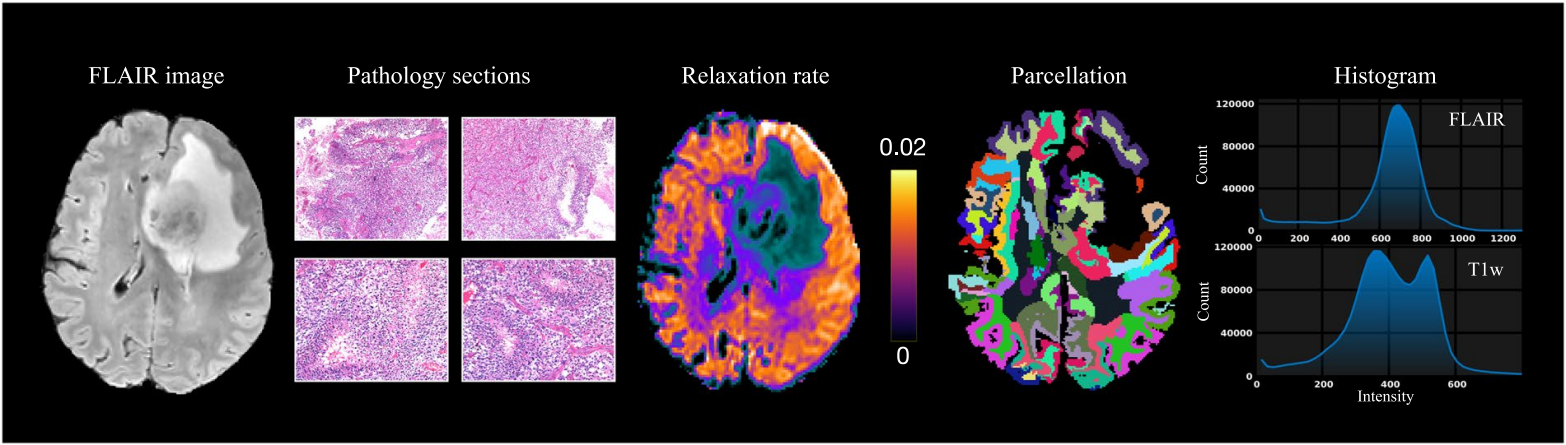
FLAIR imaging, pathology sections, and derivatives results include relaxation rate estimated by^9^, cortical parcellation generated by Freesurfer, and intensity distribution.
